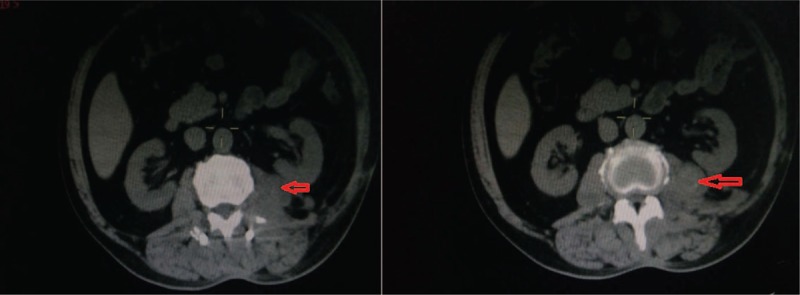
After 5 months, CT examination showed that the left lesion of the vertebral body appeared again (as arrow heads).
